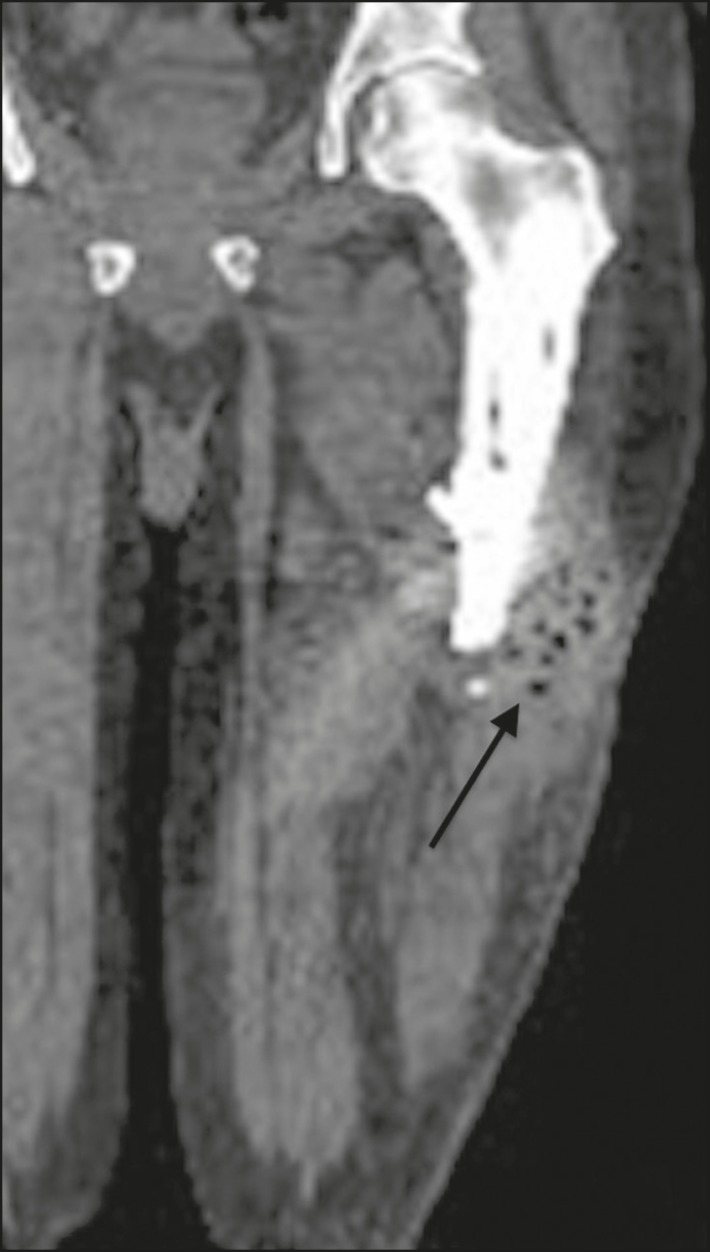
Coronal computed tomography scan of a patient with an infected left femoral prosthesis. Oval formation (arrow), measuring 9.1 × 2.3 cm, containing gas bubbles, located in the middle third of the left thigh, in contact with the femoral stem of the prosthesis, suggestive of abscess.
